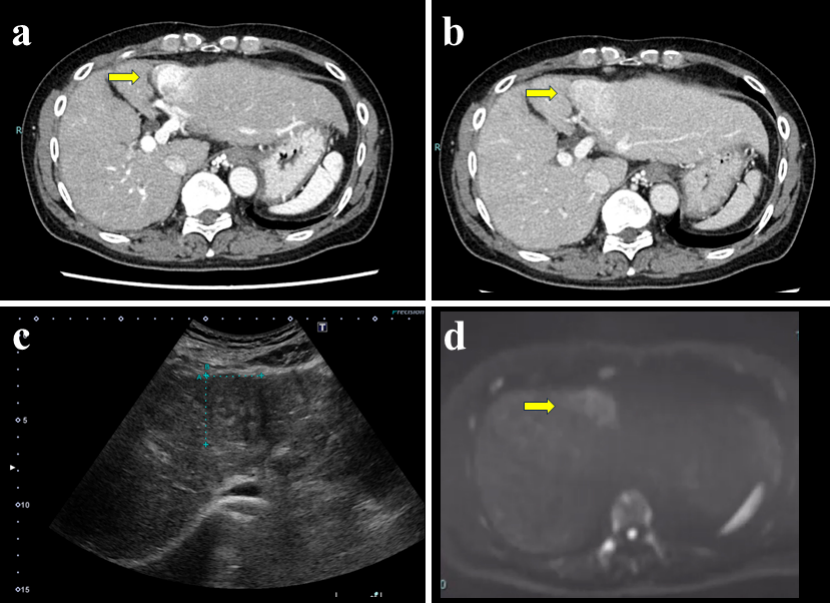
(a) Contrast-enhanced computed tomography images showing an early enhancement tumor at segment 3; the liver shape suggested a cirrhotic pattern. (b) Washout on delayed phase; (c) abdominal ultrasound showing mosaic architecture; (d) magnetic resonance imaging showing high signal intensity on diffusion-weighted images.
